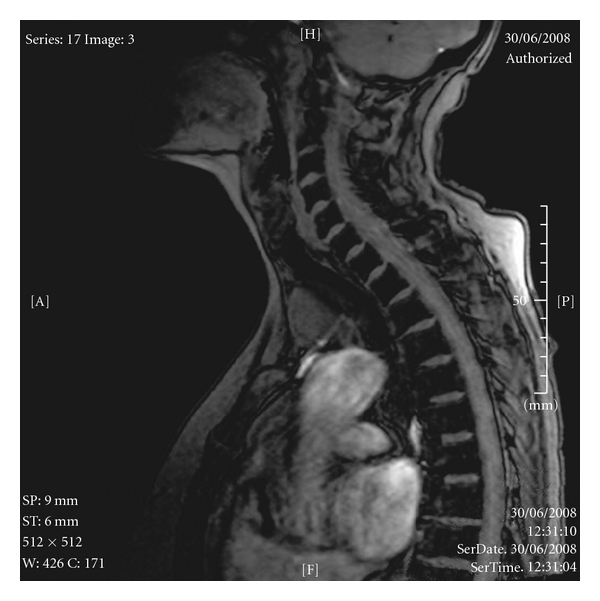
Lateral magnetic resonance image.
